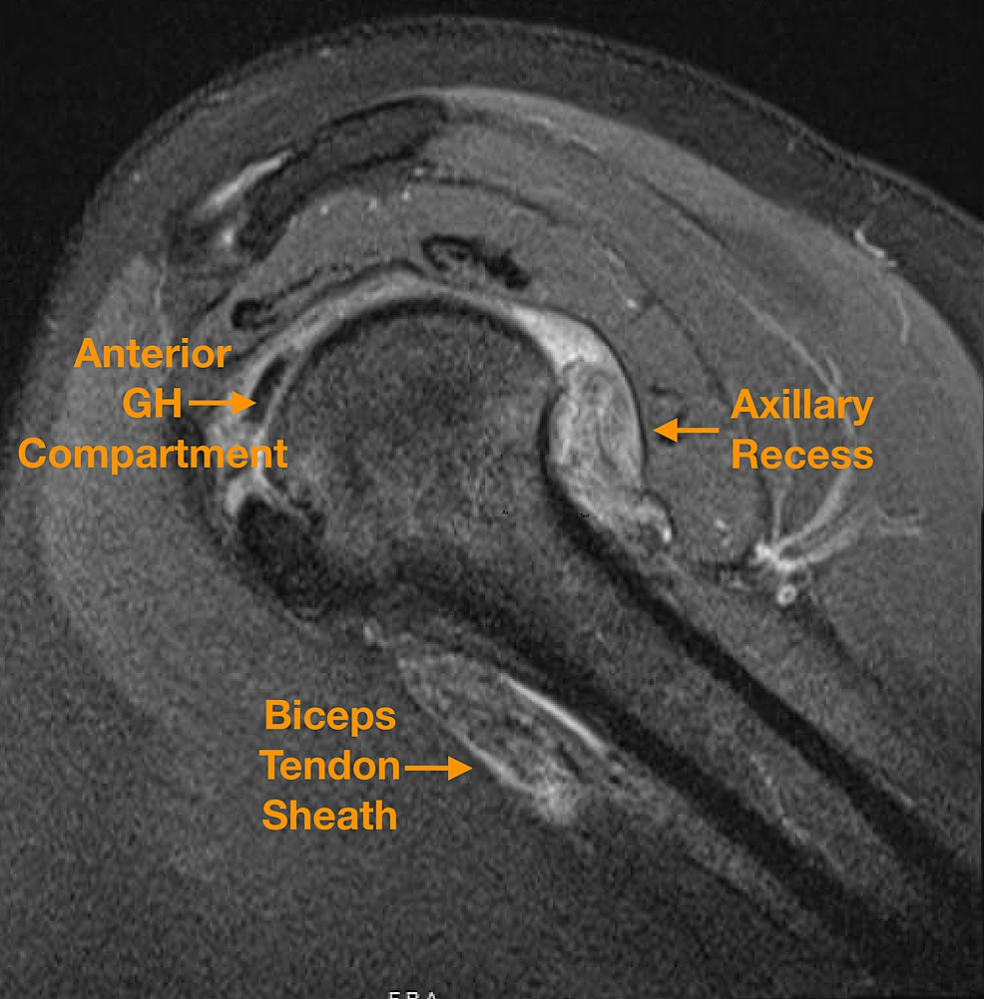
Sagittal proton-density fat-saturated MRI sequences demonstrating an intermediate-intensity, permeative, lobular lesion within the anterior glenohumeral (GH) compartment, axillary recess, and biceps tendon sheath.
